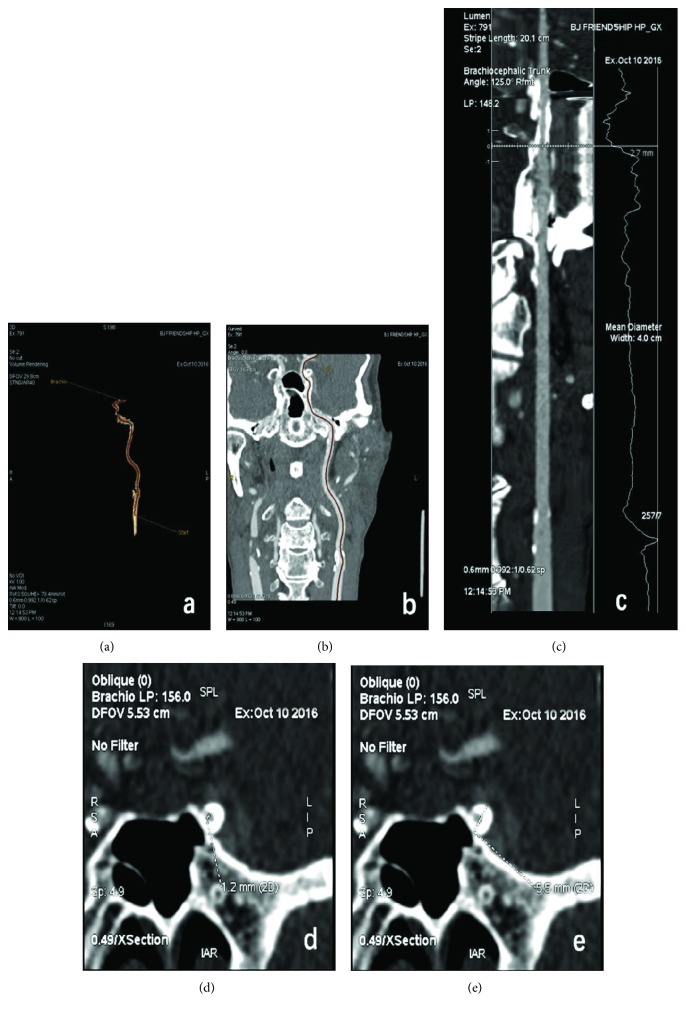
Head and neck CTA inspection. (a, b) The ICA trajectory. (c) Five segments of ICA blood vessels were measured in this study, and the measurement data of the narrowest blood vessel was selected. (d) shows the narrow vessel diameter, and (e) shows the normal vessel diameter; vascular stenosis degree % = normal vessel diameter − narrow vessel diameter, divided by the normal vessel diameter × 100%.
